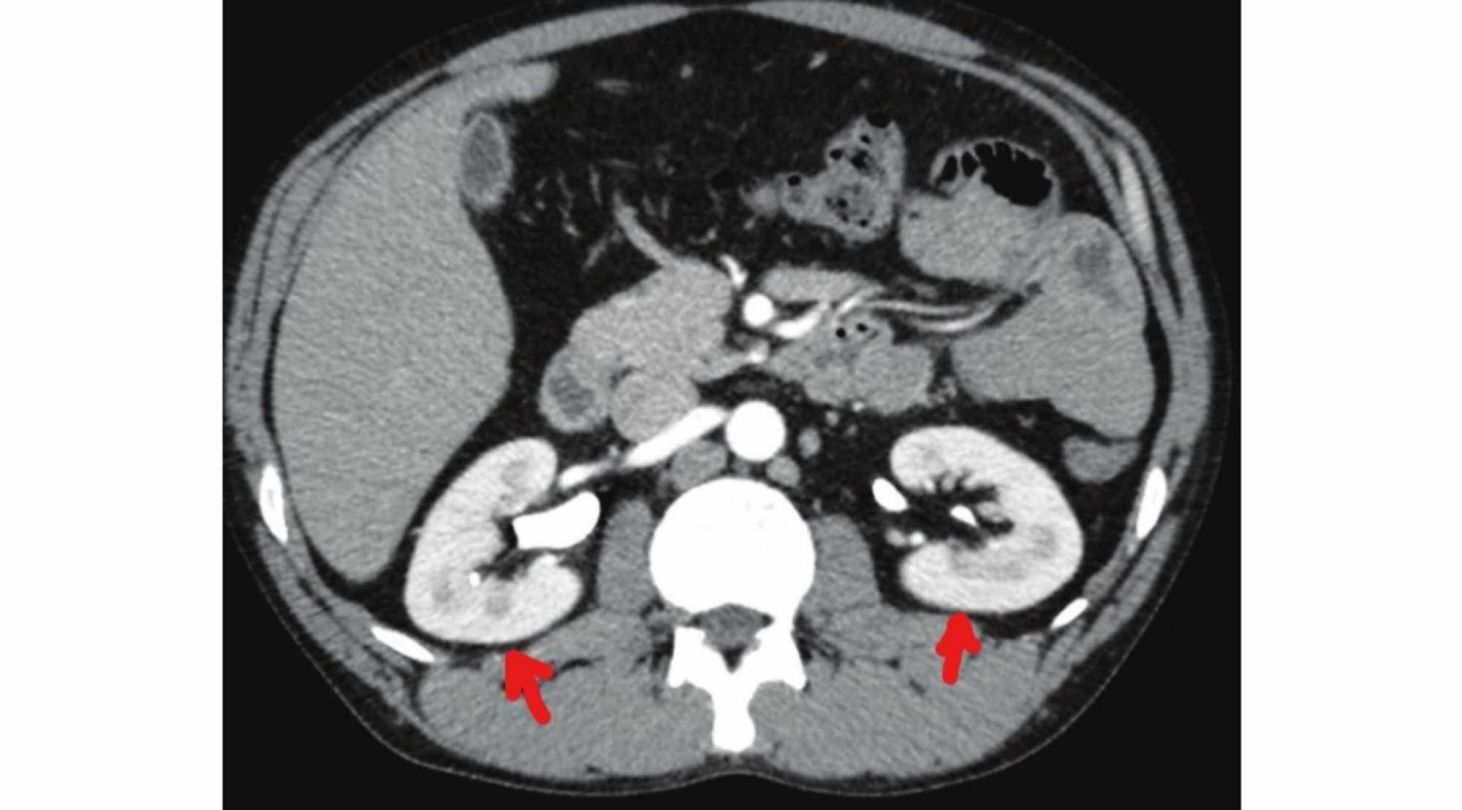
Abdominal CT Scan Study without major changes for liver, gallbladder, pancreas, and spleen, with kidneys of regular and symmetrical dimensions
